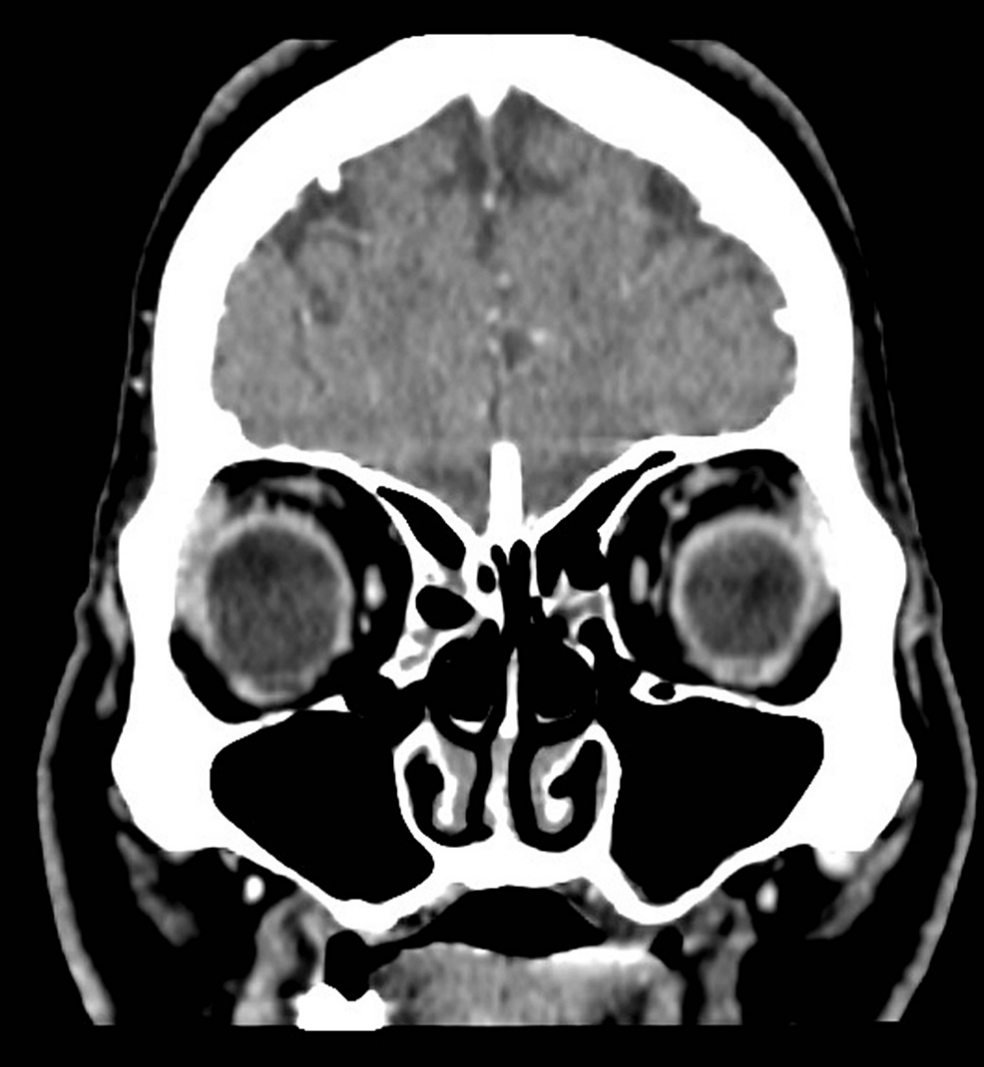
CT scan of the paranasal sinuses with contrast in the coronal plane The image shows clear sinuses including bilateral ethmoidal and maxillary sinuses.
